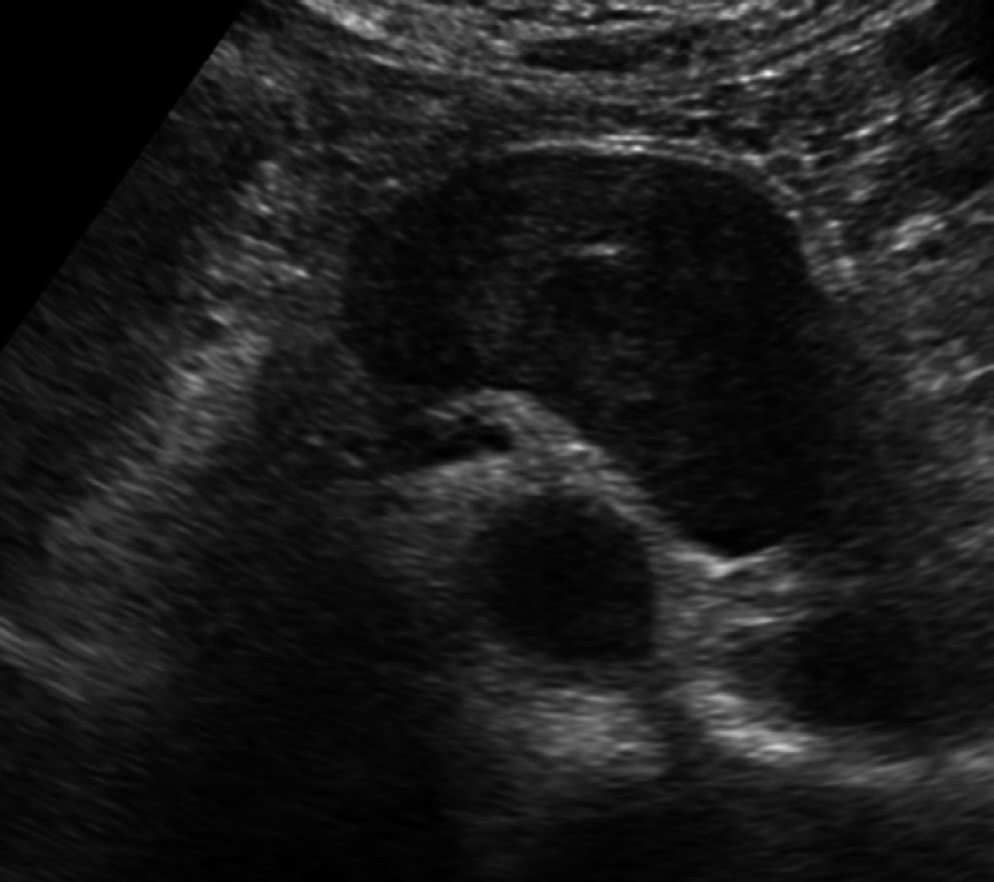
Abdominal ultrasonography image. A 56 × 43‐mm hypoechoic tumor with a smooth margin, internal heterogeneity, and no blood flow signal at the posterior wall of the gastric corpus and tail of the pancreas
